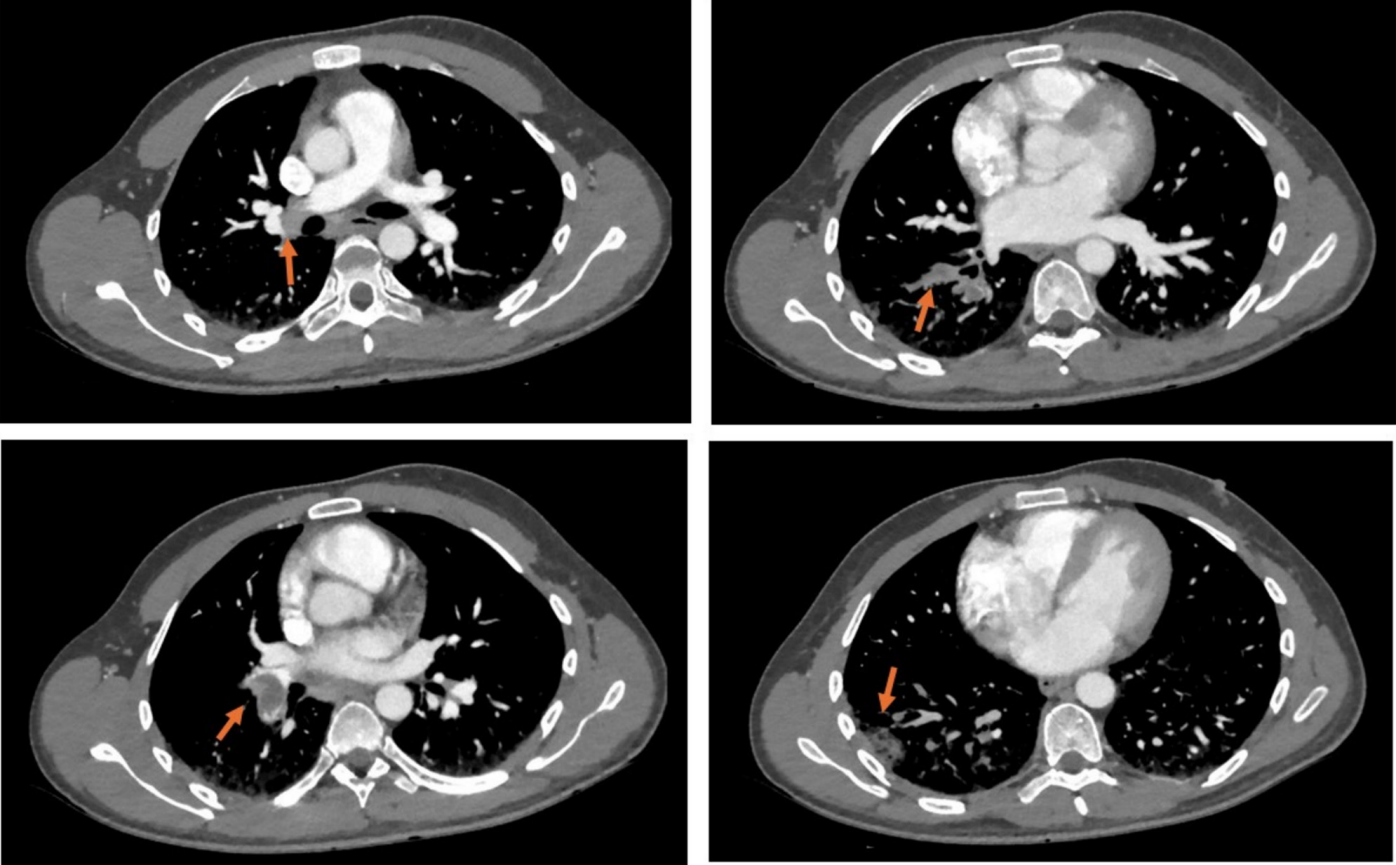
Thoracic CT angiography: Pulmonary embolism involving the right lobar branch, and signs of impaired ventilation consistent with pulmonary infarction (red arrows).
